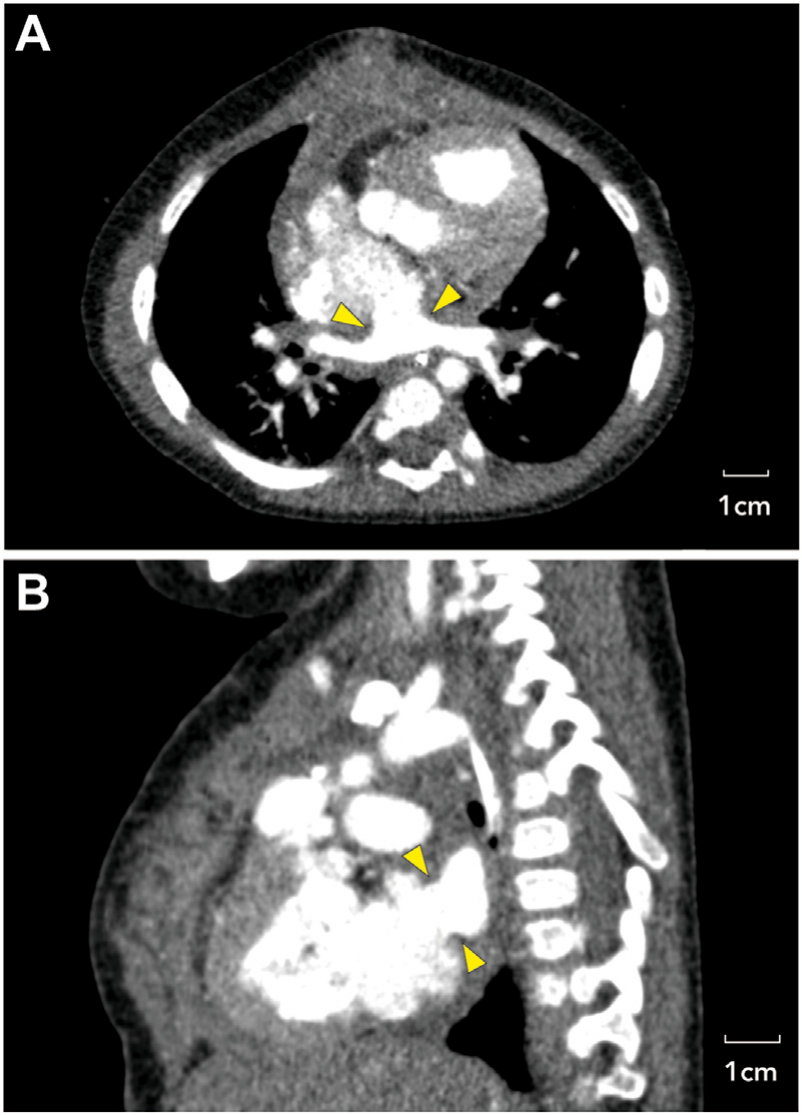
Computed tomography images taken 1 month postoperatively. (A) Axial image and (B) sagittal image showing widely patent connection between the pulmonary venous confluence and the left atrium (arrowheads).
